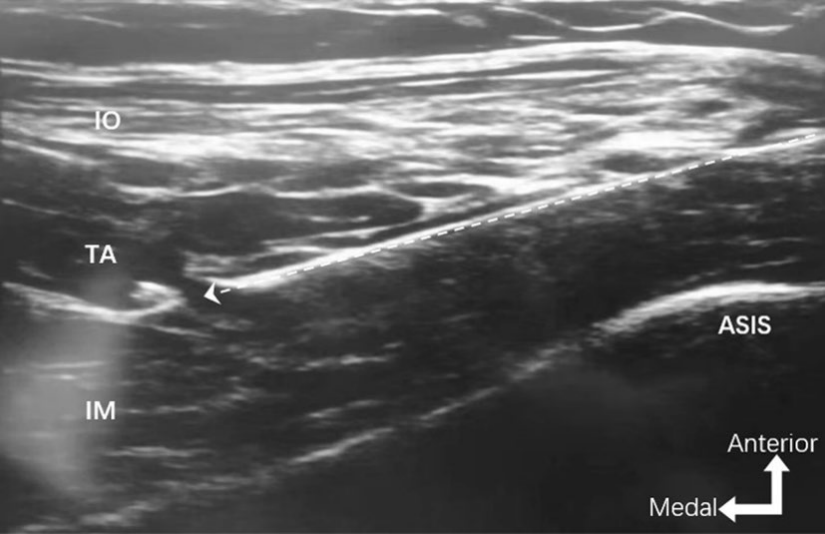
Images of ultrasound-guided supra-inguinal fascia iliaca block (S-FICB). The needle tip was located under the fascia iliacus between the transversal abdominus muscle and iliac muscle. Dashed arrow, needle pathway. IO internal oblique muscle, TA transverse abdominus muscle, IM iliacus muscle, ASIS anterior superior iliac spine
